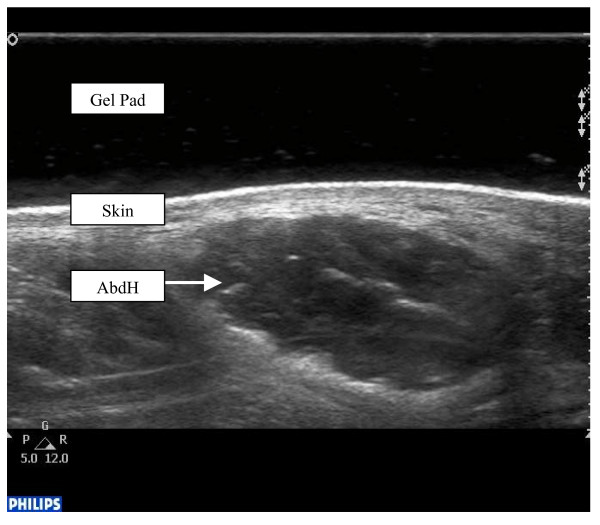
Ultrasound image of abductor hallucis muscle.
